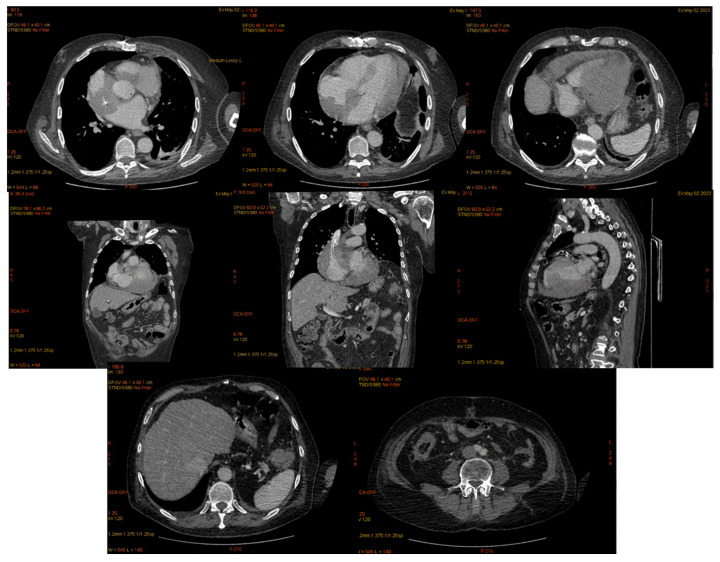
Cardiac computed tomography.
